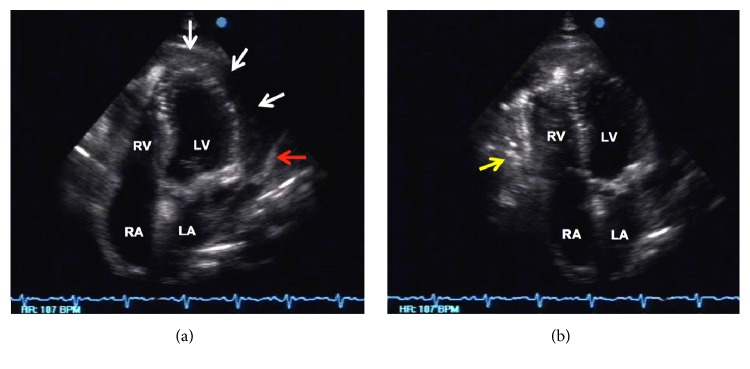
Echocardiographic apical four-chamber views demonstrating pneumopericardium. (a) There was a large (20 mm) circumferential layer (white arrows) within the pericardial space, with its heterogenous nature suggestive of possibly purulent pericardial fluid. Marked thickening of the pericardium (5 mm) can also be appreciated in this view (red arrow). (b) Slight angulation of the probe to focus on the anterior pericardial space (anterior to the right ventricle) revealed the presence of microbubbles (yellow arrow) representing air freely moving within the pericardial sac.
